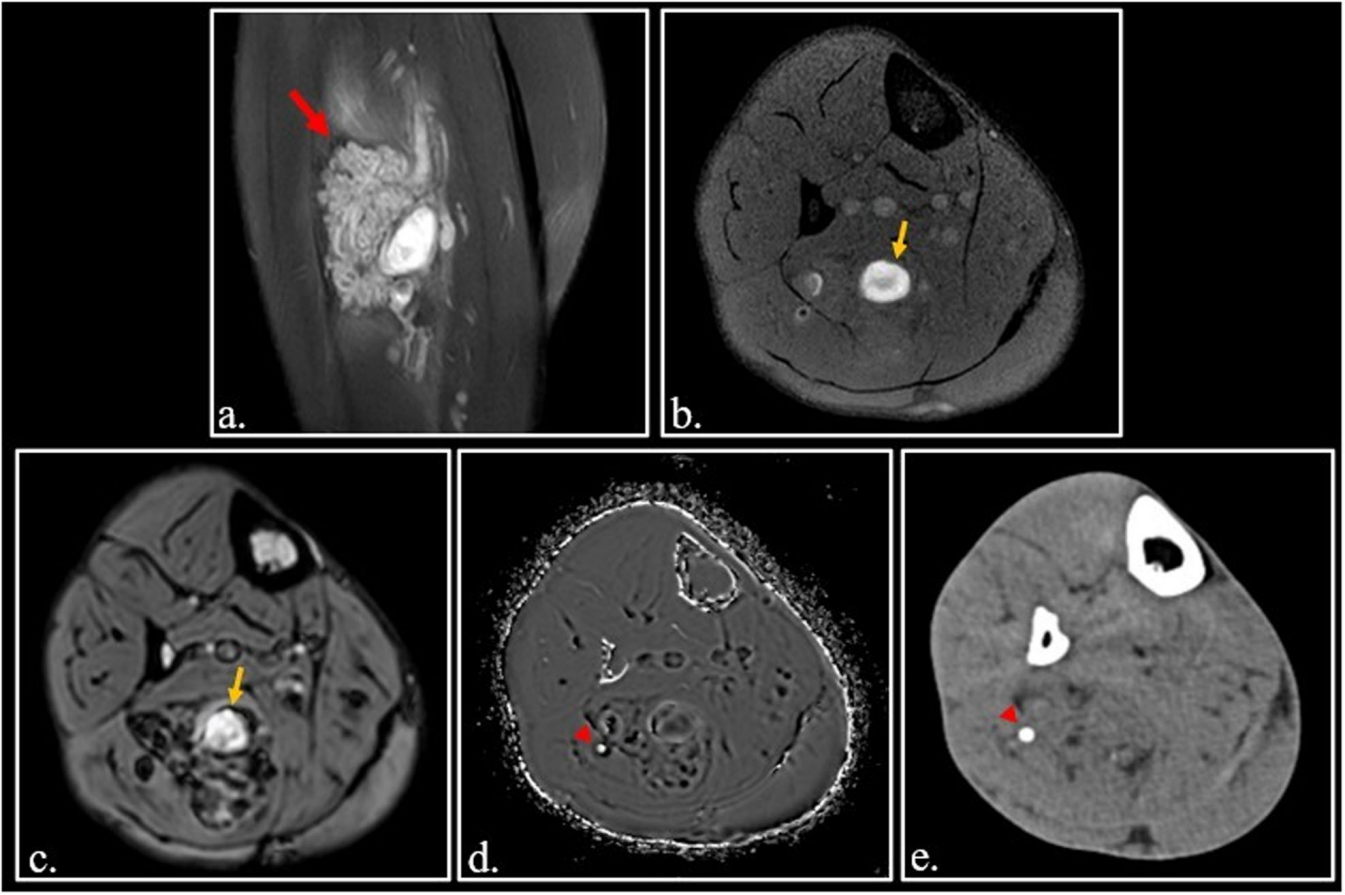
Sagittal FSE PD-weighted fat-sat (a) and Axial FSE T1-weighted (b) images in a 25-year-old male with muscular slow-flow malformation of the right calf (red arrow in (a)). An intralesional hyperintense hematoma is visible on the T1-weighted image (orange arrowhead). On SWI (c), the hematoma appears hyperintense (orange arrow). It is surrounded by multiple hypointense spots due to intracellular deoxyhemoglobin into vessels. On phase map (d), a white spot due to a calcified phlebolith (arrowhead) can be detected at the margin of the lesion. CT scan examination (e) confirms the presence of a calcified phlebolith (arrowhead)
